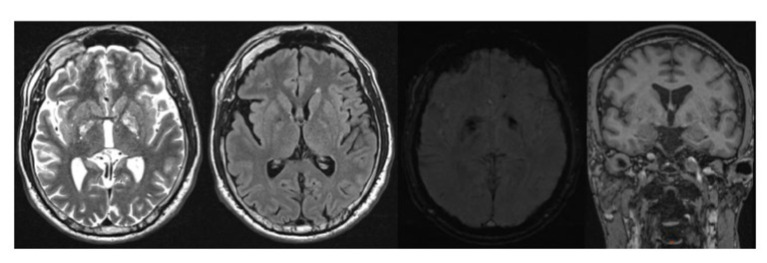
MRI Brain T2 weighted, FLAIR, SWI, T1 weighted images showing mild generalized cortical atrophy and iron deposits in the bilateral globus pallidus.
